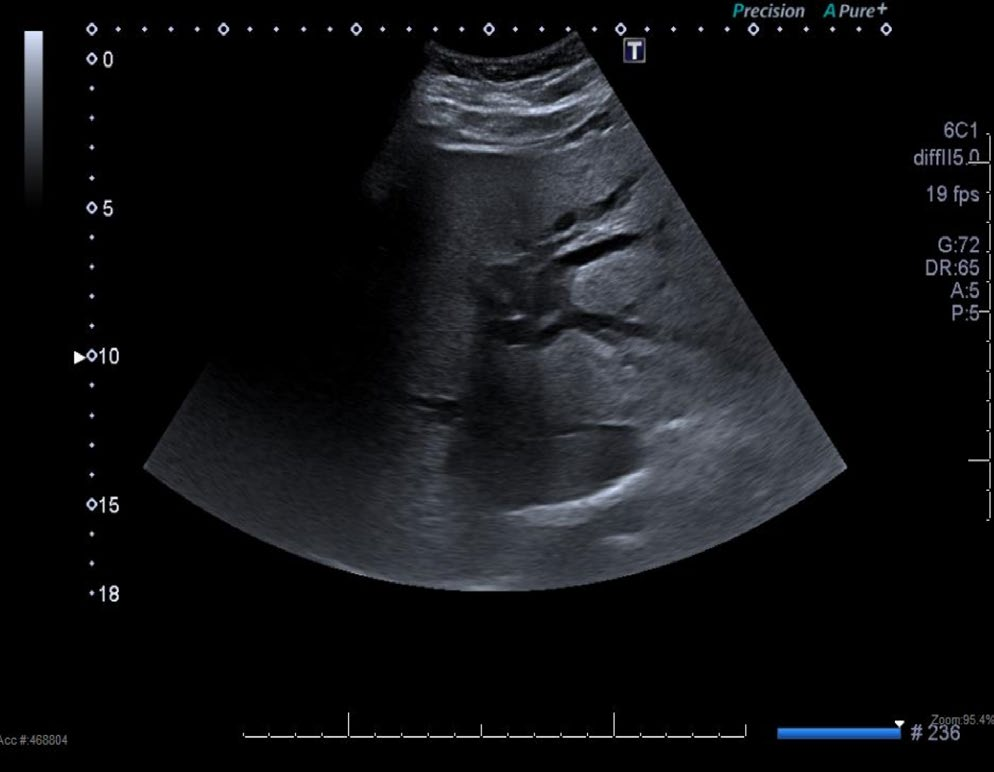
Follow‐up abdominal ultrasound demonstrating persistent dilatation of the intrahepatic bile ducts.
